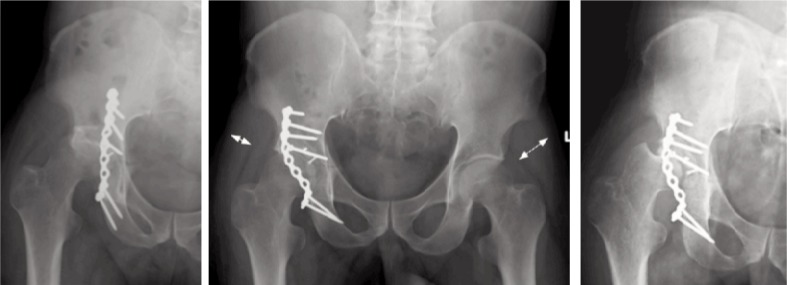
Radiographs at one month showing lateral subluxation, after revision surgery, and at 5 months showing persisting subluxation. Note the wasting of the gluteus medius (white arrow) compared to the contralateral hip (dotted arrow).
